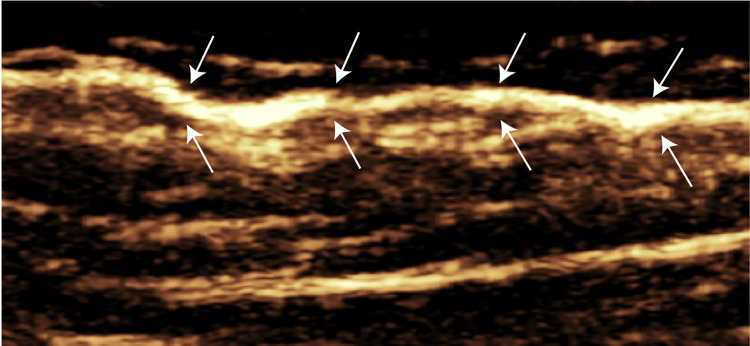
Contrast-enhanced ultrasound image from a healthy volunteer. AArrowss indicate the lymphatic vessel.
